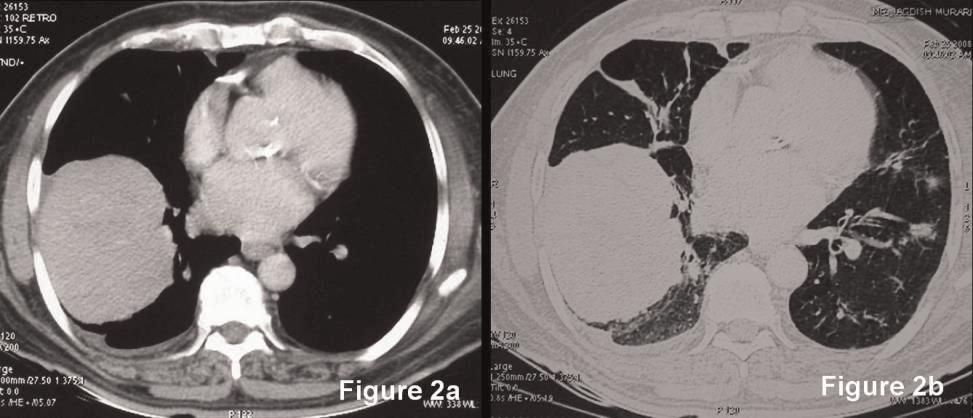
(a) and (b) Contrast enhanced CT scan of the chest. Contrast enhanced CT of chest (both mediastinal and lung windows) showing a 9.8 cm × 7.6 cm, homogenous pleural based mass in right lobe of lung and mild right pleural effusion. Multiple varying size nodular lesions involving left upper lobe and apical segment of left lower lobe suggestive of metastatic lung disease.
